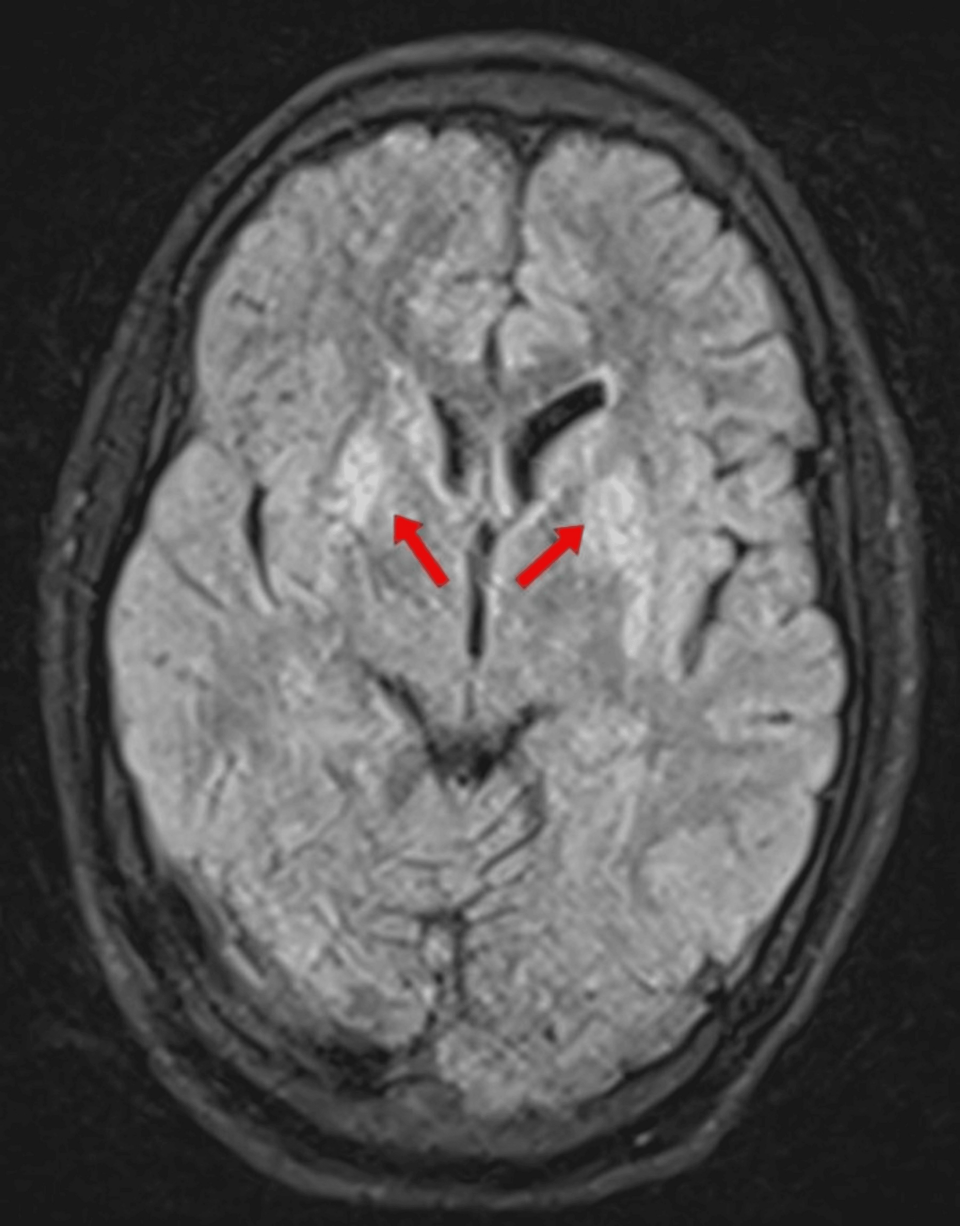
MRI brain of the middle sibling MRI, Magnetic Resonance Imaging; FLAIR, Fluid attenuated inversion recovery; T2-FLAIR showing bilateral basal ganglia hyperintensity.
